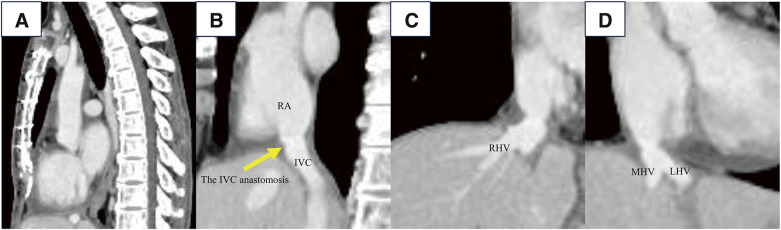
Computed tomography images at 3 months postoperatively. (A): sagittal section. (B–D): coronal sections. (A) The computed tomography scan performed 3 months postoperatively revealed no findings within the mediastinum, and (B–D) no thrombi were detected in the IVC or major hepatic veins. IVC, inferior vena cava; LHV, left hepatic vein; MHV, middle hepatic vein; RA, right atrium; RHV, right hepatic vein
